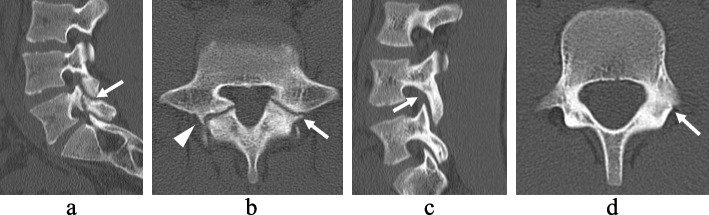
Computed tomography images of L5 and non-L5 spondylolysis. L5 spondylolysis (a, b). Bilateral lesions are present, the right lesion is a pseudarthrosis with osteosclerosis (arrowhead) and the left lesion is a progressive stage with a clear gap (arrow). Non-L5 spondylolysis (c, d). Unilateral lesion in L4. Early-stage lesion with hairline (arrow) on the left side only
